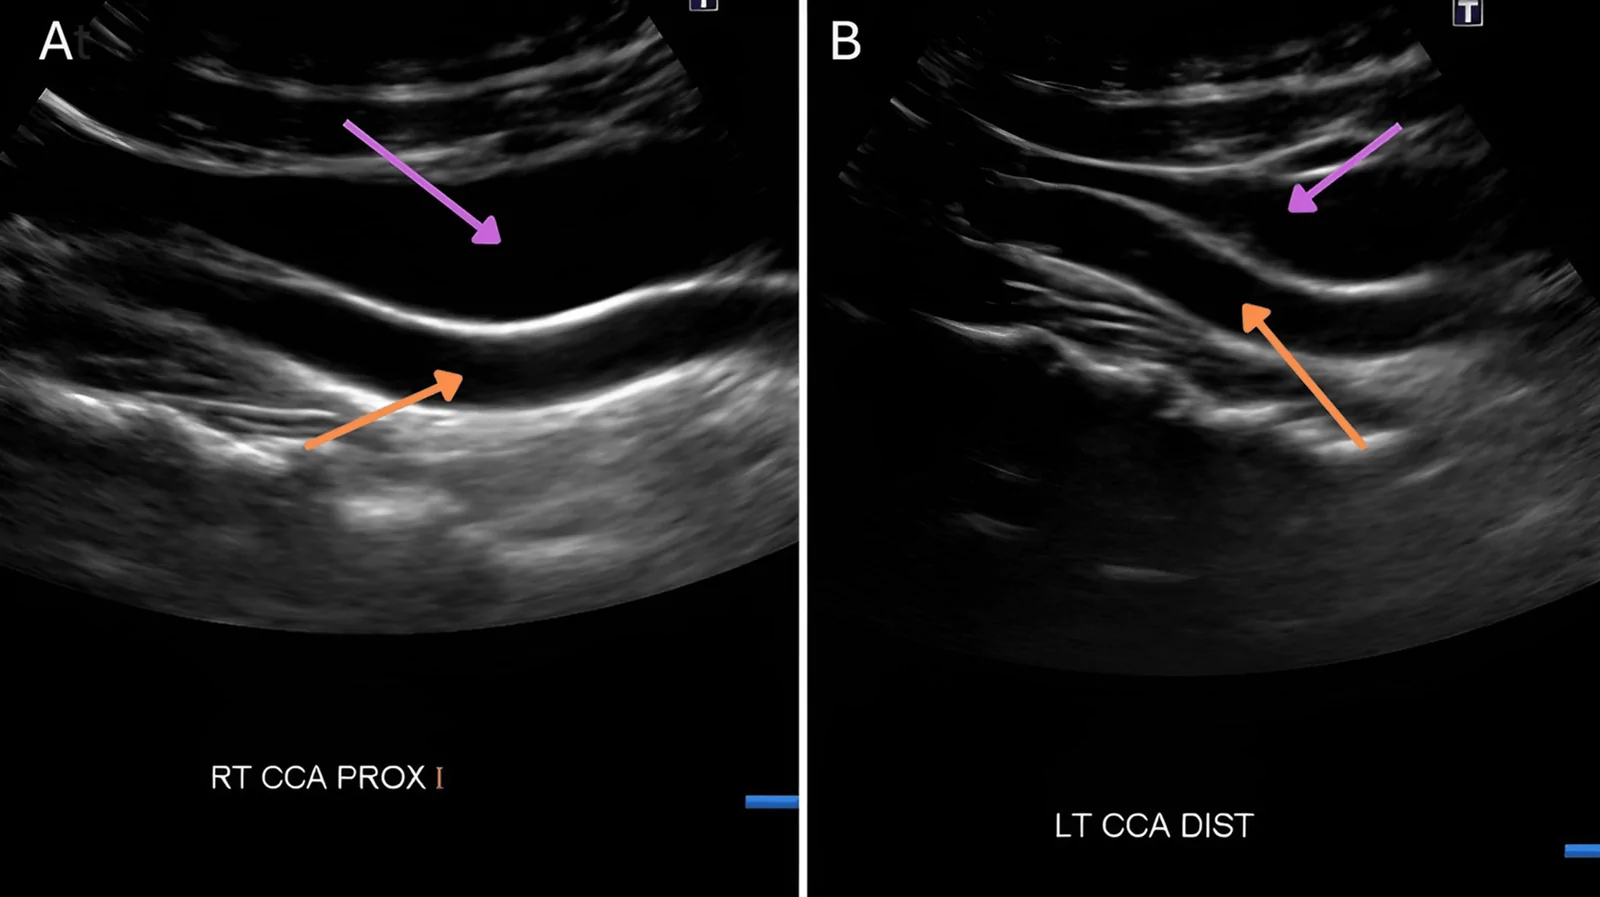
Gray-scale ultrasound images of the right proximal (A) and left distal (B) common carotid arteries demonstrating mild (<50%) stenosis with preserved luminal patency. The common carotid artery (orange arrows) and adjacent internal jugular vein (purple arrows) are labeled for orientation RT: right; LT: left; CCA: common carotid artery; PROX: proximal; DIST: distal
